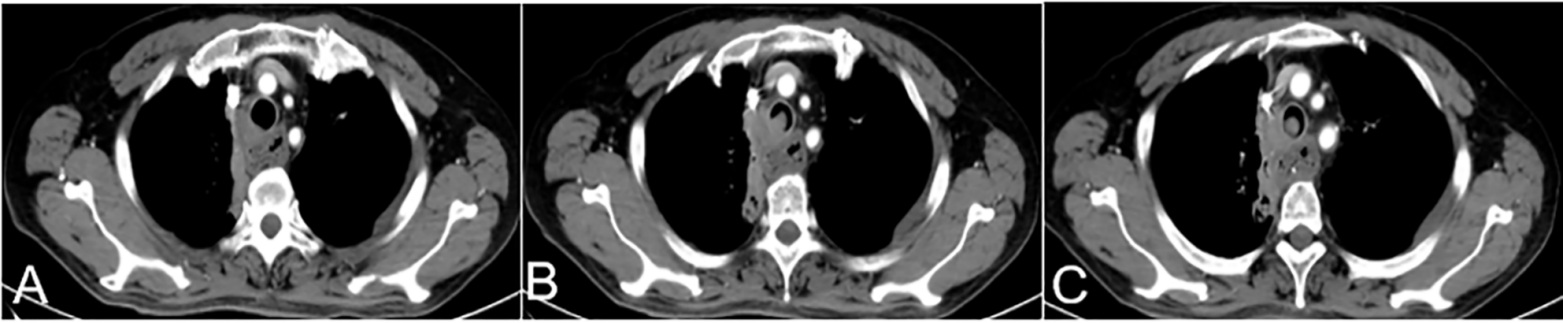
A 63-year-old female with hemoptysis and dyspnea more than half a month after esophageal cancer surgery. (A–C) Preoperative CT showed a soft tissue density shadow on the right side of the trachea at the thoracic entrance, protruding into the trachea. This soft tissue density shadow corresponded to stenosis of the middle trachea, which was evenly enhanced CT, computed tomography.
